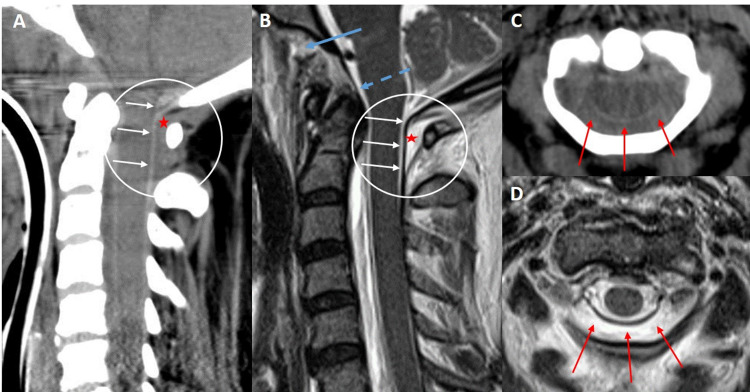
40-year-old female status post motor vehicle accident presenting for cervical CT and MRI exam. Sagittal CT (A) and T2 weighted MRI (B) of the craniocervical junction demonstrate a stripping injury of the posterior dura and posterior atlanto-occipital membrane from the posterior C1 arch (white arrows) with a localized posterior epidural hematoma at C1-C2 (red stars). Associated tears of the anterior atlanto-axial membrane (blue arrow) and a stretch injury of the tectorial membrane (blue dashed arrow) are also present. Axial CT (C) and T2 weighted MRI (D) images of the same patient demonstrate the posterior epidural hematoma between the posterior dura and posterior C1 arch. CT: computed tomography, MRI: magnetic resonance imaging
